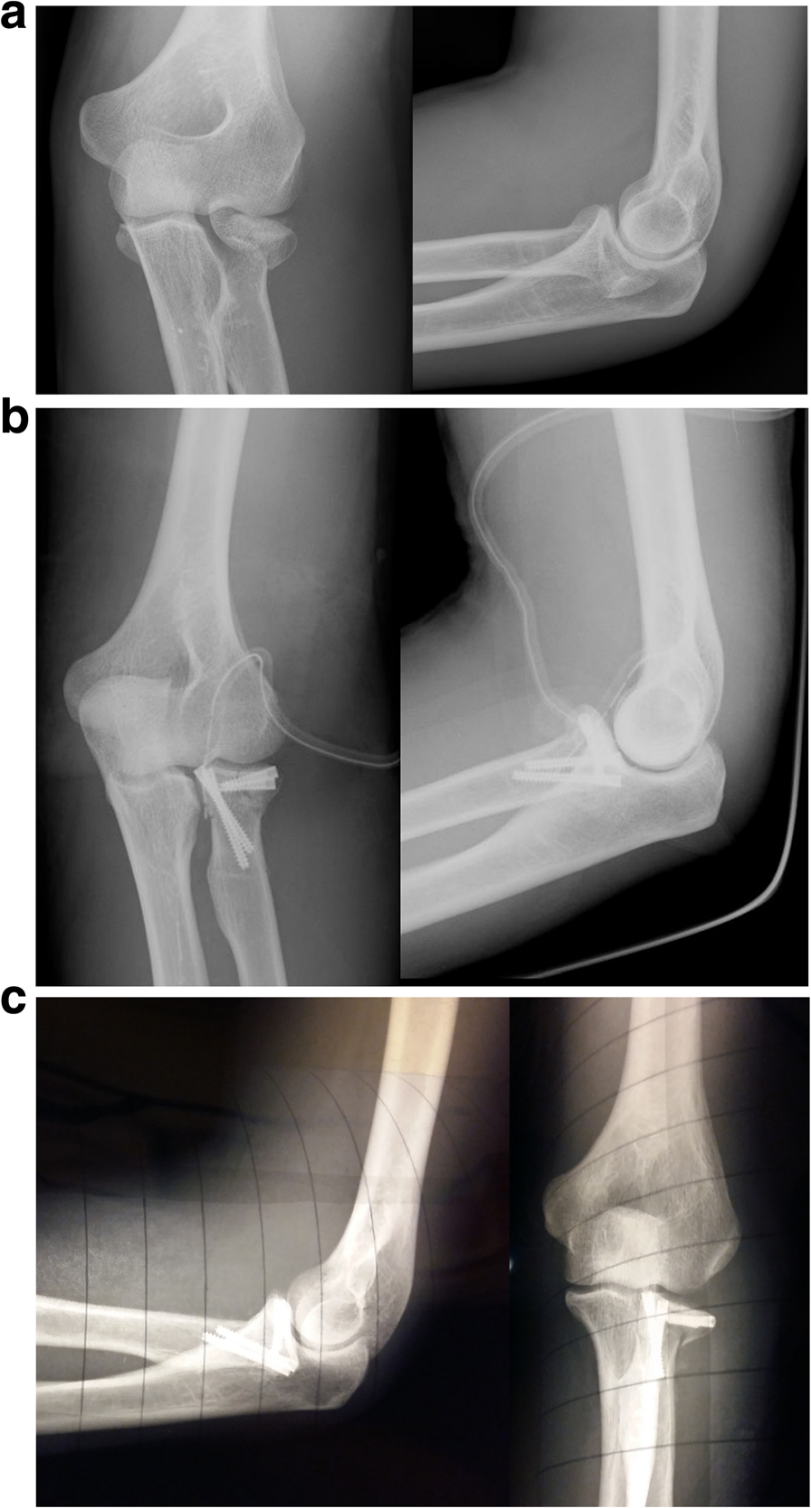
a X-rays showing a Mason type III fracture. b Post-operative x-rays with acceptable reduction using mini-screws. c X-rays showing secondary displacement of fracture fragments of about 4 mm observed 1 months after surgery
